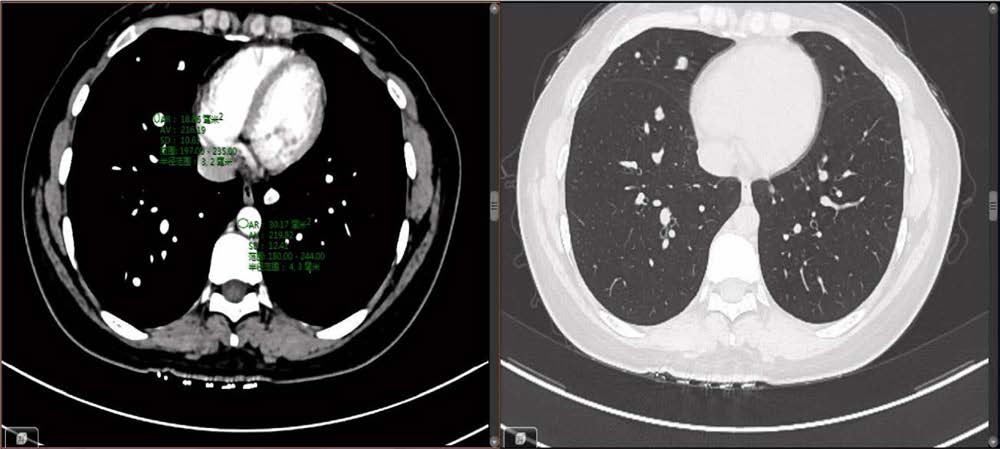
The CT presentation of lung metastases in this patient showed the same CT values as the vascular CT values. CT = chest computed tomography.
